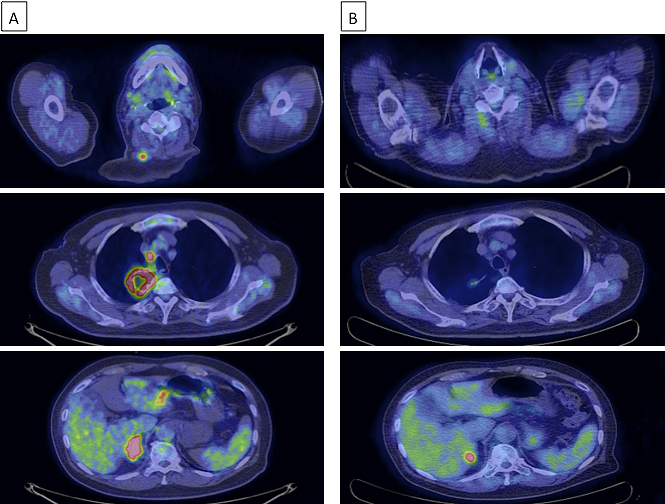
Images from positron emission tomography (PET)-CT scan of the patient. A. Fluorodeoxyglucose uptake in the mediastinal lymph node, right adrenal gland, and right trapezius muscle. B. A significant decrease in lung and metastatic tumor size as indicated by reduced fluorodeoxyglucose uptake, 21 weeks after treatment began.
